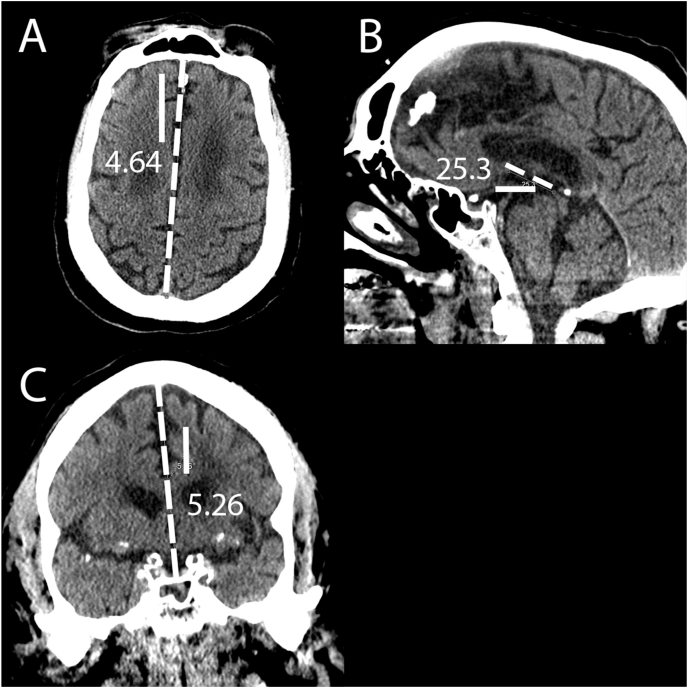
Manual whole head alignment validation measurement technique. CT images are shown in a soft tissue window setting to better demonstrate measurement landmarks on this unaligned head CT. (A) Axial alignment (degrees angulation from the dashed line between the anterior and posterior attachments of the falx) is off by 4.64° in the clockwise direction for a value of +5 (A). (B) Sagittal alignment (degrees angulation from the dashed AC-PC line) is off by 25.3° in the clockwise direction for a value of +25. (C) Coronal alignment (degrees angulation from the dashed line drawn between the center of the sella inferiorly and superior sagittal sinus superiorly) is off by 5.26° in the counter-clockwise direction for a value of −5 (C).
